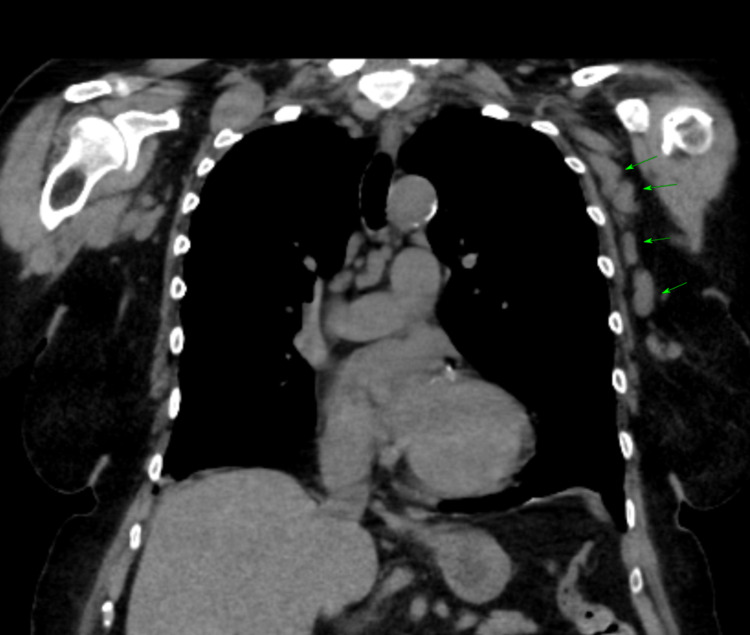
HRCT chest showing enlarged left axillary lymphadenopathy (green arrows) HRCT: High-Resolution Computerized Tomography
